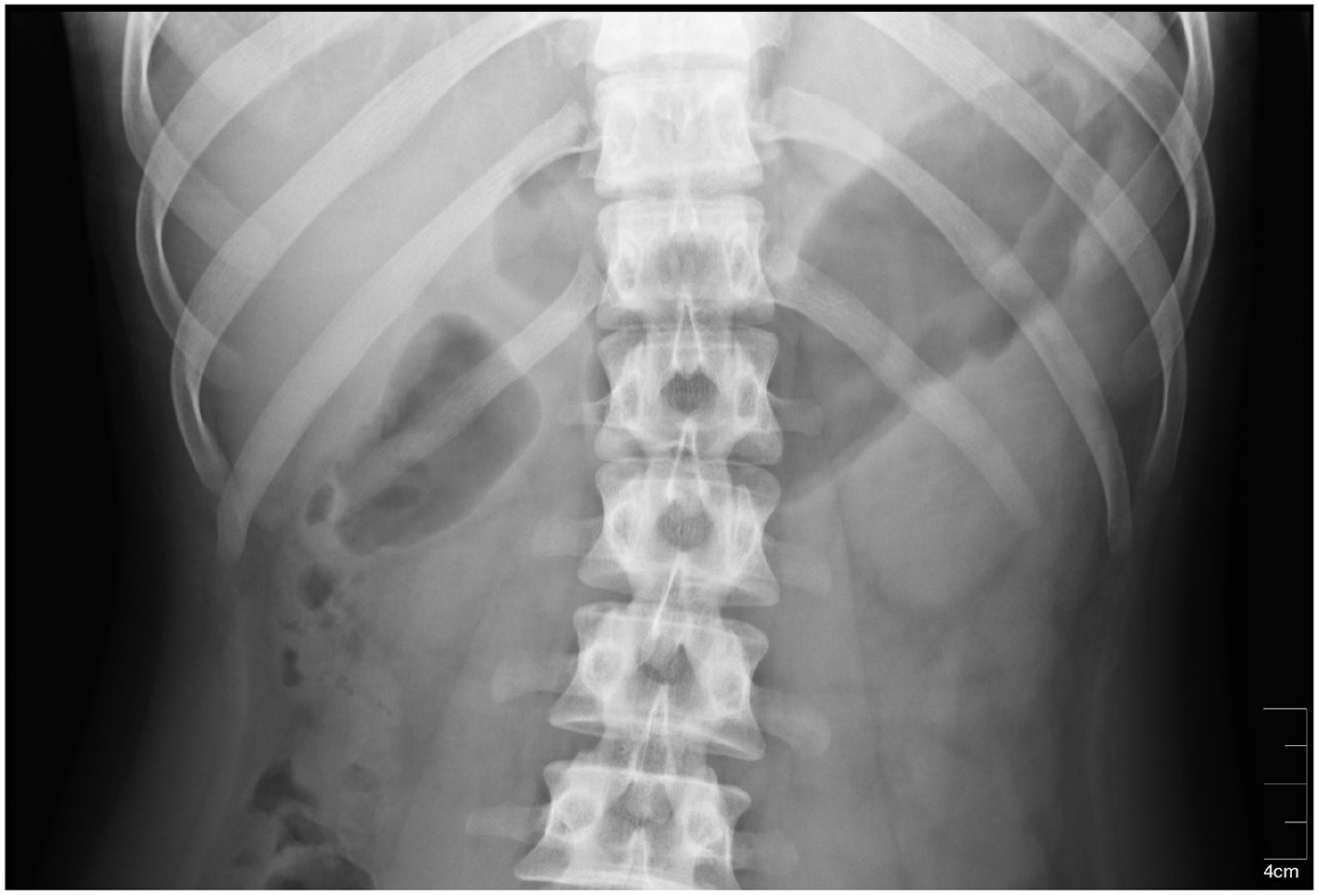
An X-ray image of the stomach. The stomach is located in the dark area on the right side of the radiograph (51).
